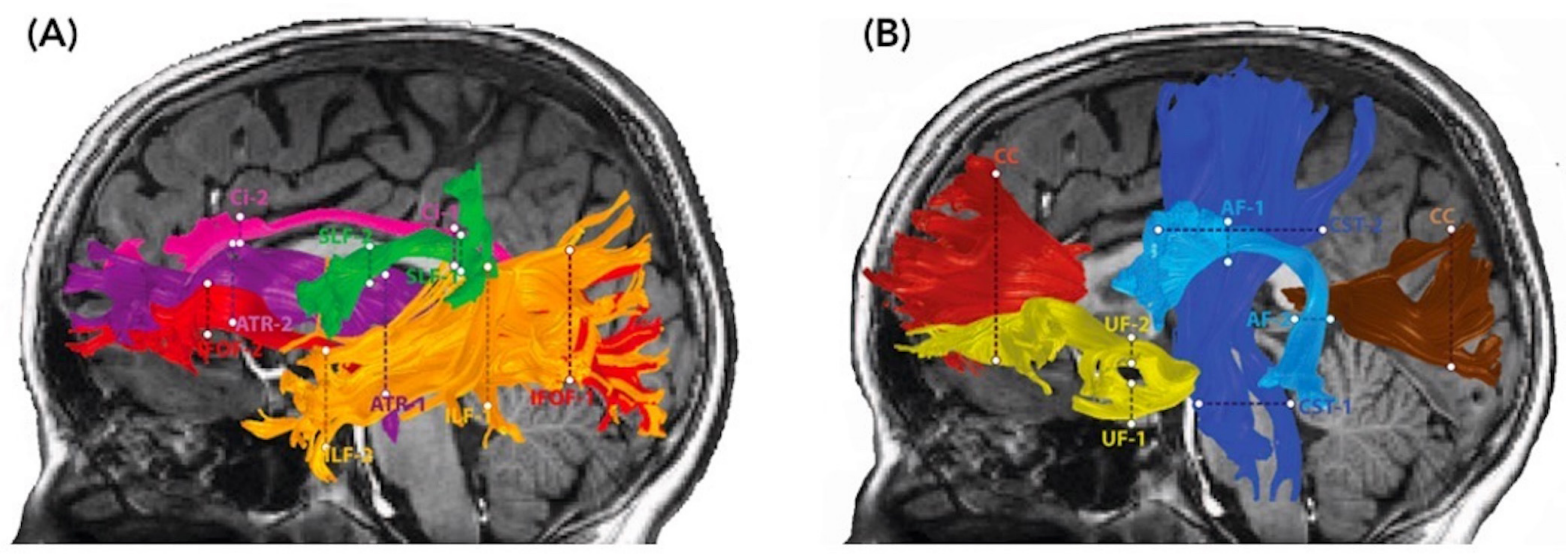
Diffusion MRI tractography of cerebral white matter pathways. Renderings of 10 major white matter pathways are overlaid on two sagittal T1-weighted images of a representative child with NF1 (age 8.5 years, male). The defining waypoint regions of interest (ROIs) are marked by dotted lines. The left panel (A) depicts the anterior thalamic radiation (ATR) = purple, superior longitudinal fasciculus (SLF) = green, cingulate (Ci) = pink, inferior fronto-occipital fasciculus (IFOF) = light red, and inferior longitudinal fasciculus (ILF) = orange. The right panel (B) depicts the corticospinal tract (CST) = dark blue, uncinate fasciculus (UF) = yellow, arcuate fasciculus (AF) = light blue, corpus callosum forceps minor (CC) = dark red, and corpus callosum forceps major (CC) = maroon.
